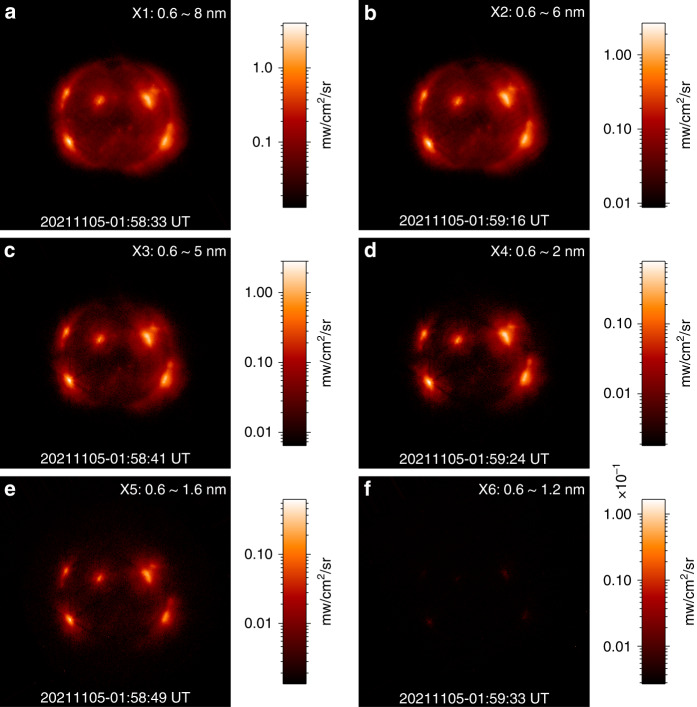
X-ray images with absolute brightness from X-EUVI. The observation time is at the bottom, and the imaging channels are at the upper right corners
